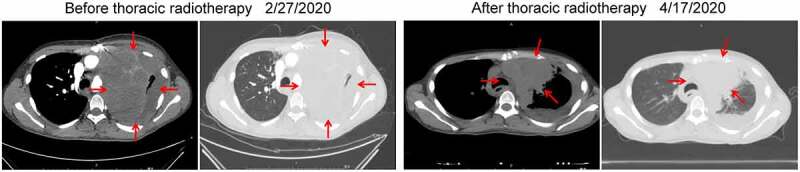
CT imaging before and after thoracic radiotherapy. After local radiotherapy, the lesions that had metastasized to the left lung significantly subsided compared with that before local radiotherapy. CT: computed tomography
